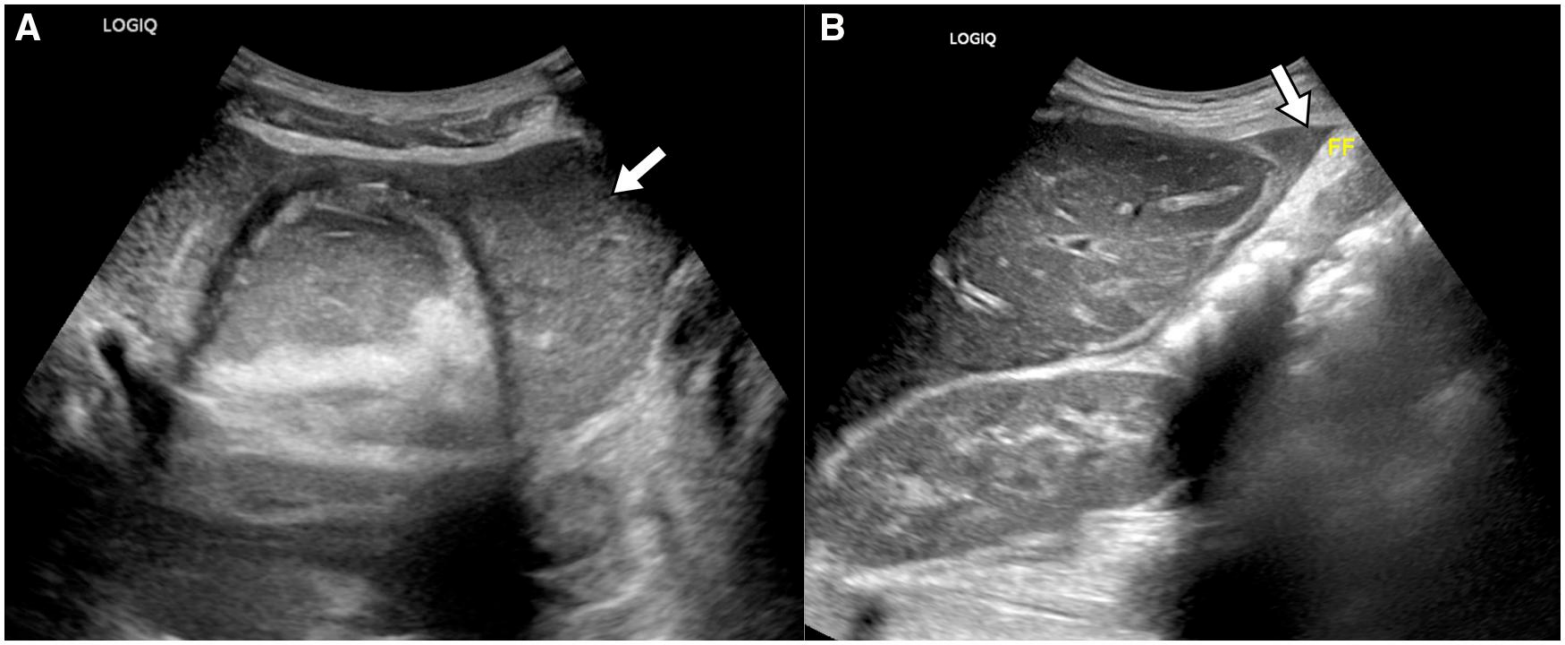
(A) Ultrasonogram of the pelvis shows echogenic fluid surrounding the left adnexal mass (arrow). (B) The echogenic fluid is also seen at the anterior surface of the liver (arrow). This echogenic fluid may represent blood or lipid laden fluid within the peritoneal cavity.
